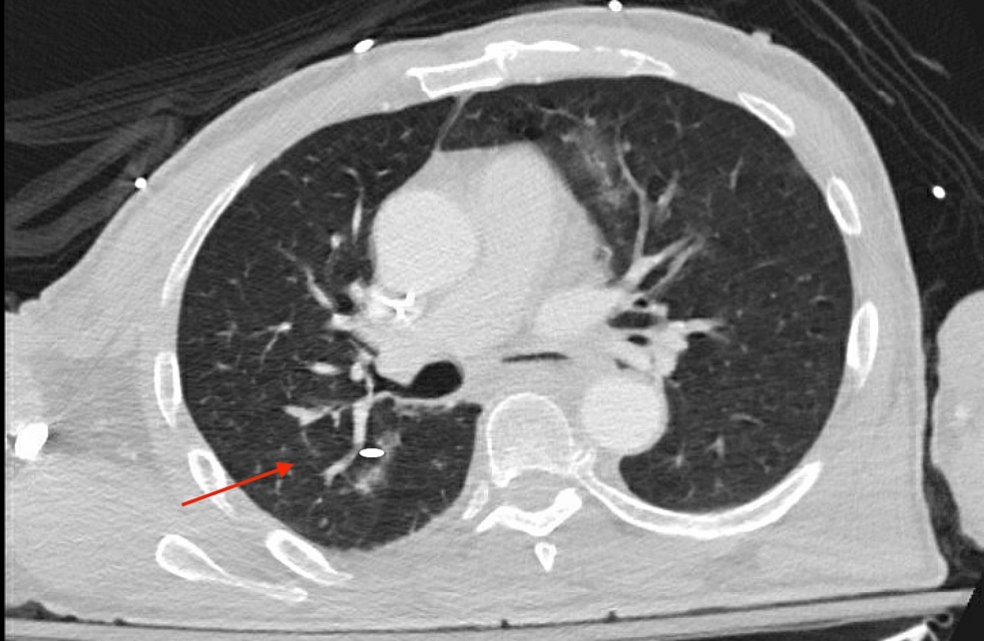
CT chest with contrast depicting multifocal alveolar disease, predominantly ground-glass opacification most severe in the left upper lobe. Mild tree-in-bud centrilobular nodularity identified in the right upper lobe (arrow)
